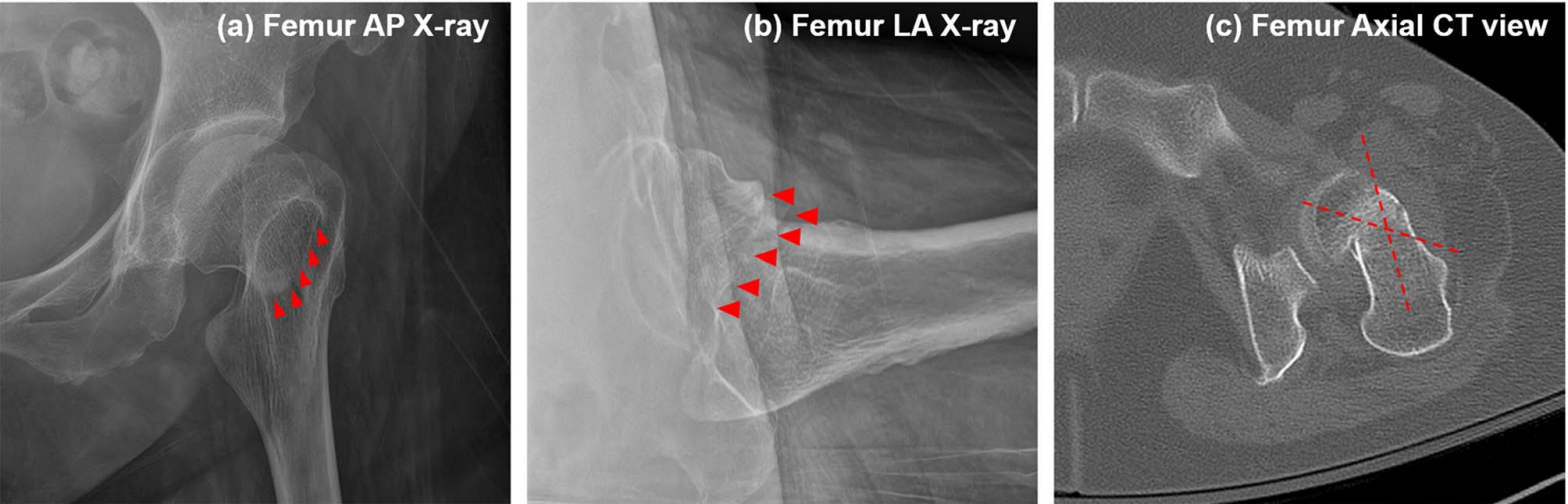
Example for misclassification of femoral neck fractures in X-ray images. Images of a 69-year-old female patient are presented: (a) X-ray anteroposterior (AP) view, (b) X-ray lateral (LA) view, and (c) axial computed tomography (CT) scan. The ensemble model classified the fracture as Garden type IV based on X-ray images of the AP and LA views. However, CT scan evaluation showed the fracture to be Garden type III due to posterior angulation.
